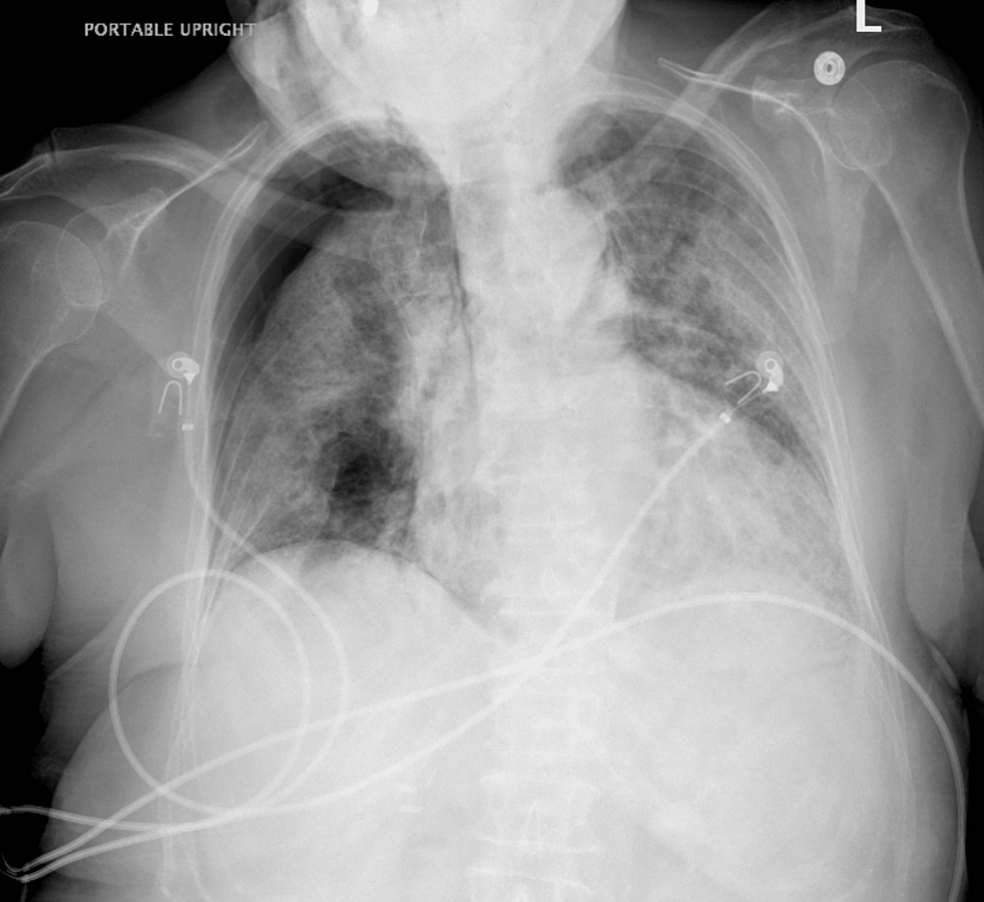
Chest radiograph showing a significant right pneumothorax.
